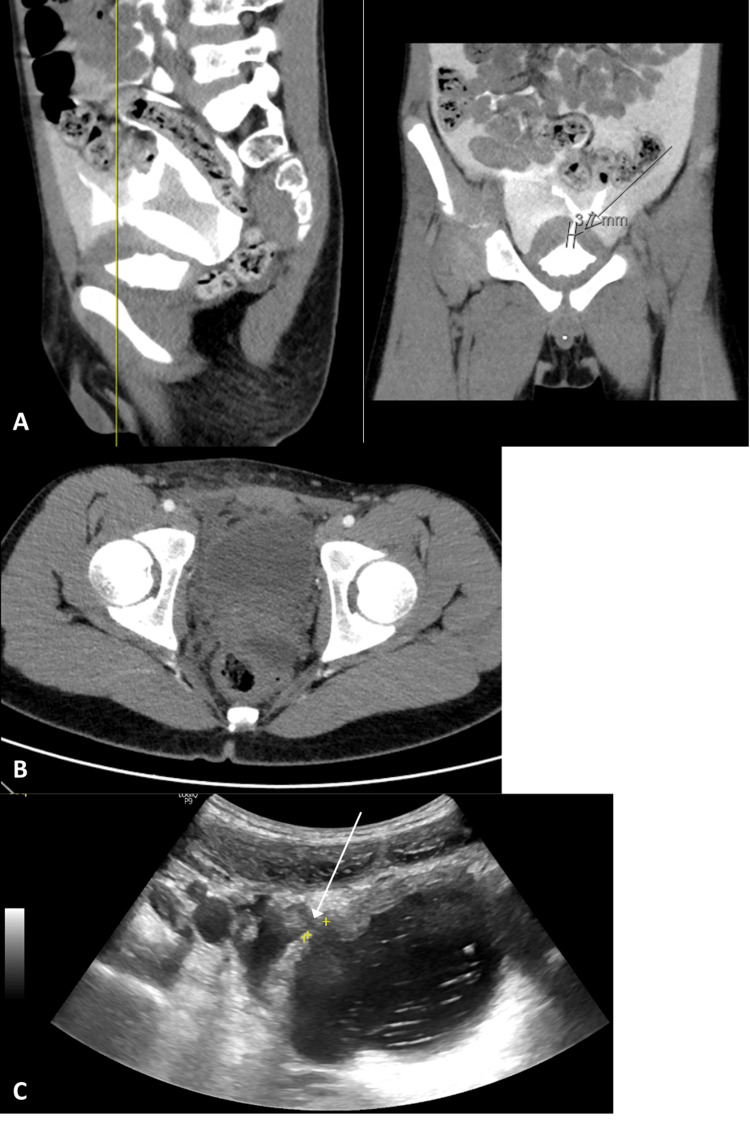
Imaging methods performed for diagnosis. (A) CT with intravesical contrast. (B) Non-diagnostic CT, without excretory phase. (C) Ultrasound with intravesical saline infusion, showing the extravasation of fluid through the rupture (arrow).
